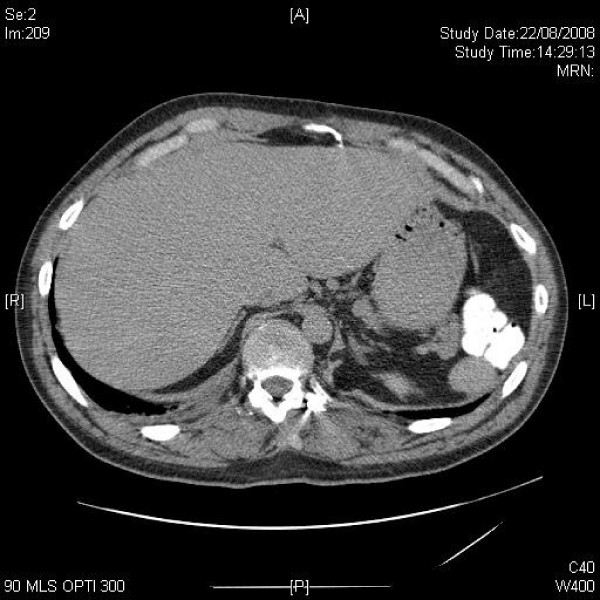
Axial CT 5 minutes post-contrast demonstrates complete homogenous enhancement of the haemangioma, now being of the same density as normal surrounding liver. There is a 3 cm splenunculus on the left side.
